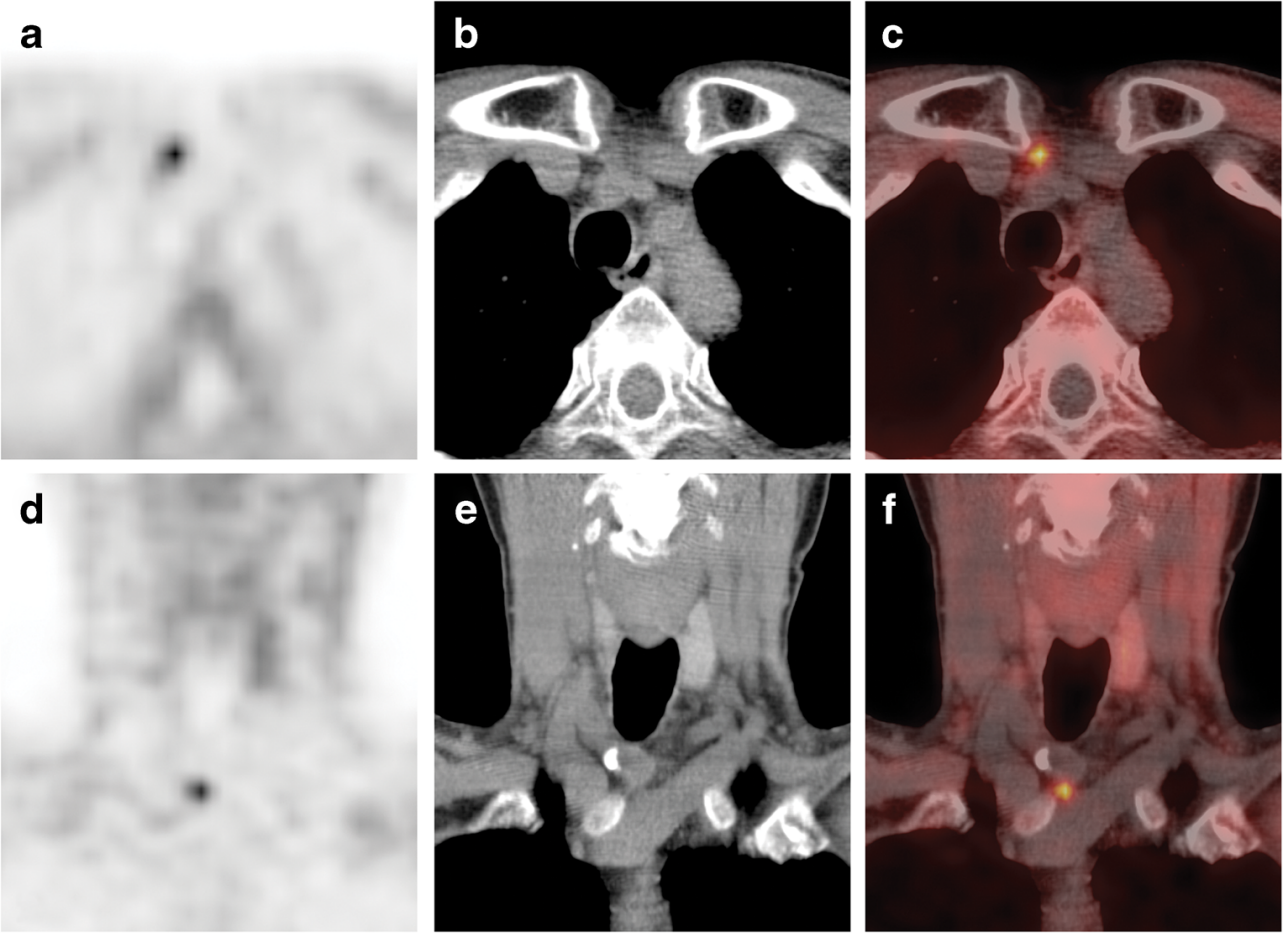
^18^F-Fluorocholine PET-CT of the neck/mediastinum: axial (a-c) and coronal (d-f) PET, CT and fused PET-CT images showing a focal uptake (SUVmax = 1.8) at the lower anterior neck, level VI, anterior to the right common carotid artery, suggesting parathyroid adenoma
